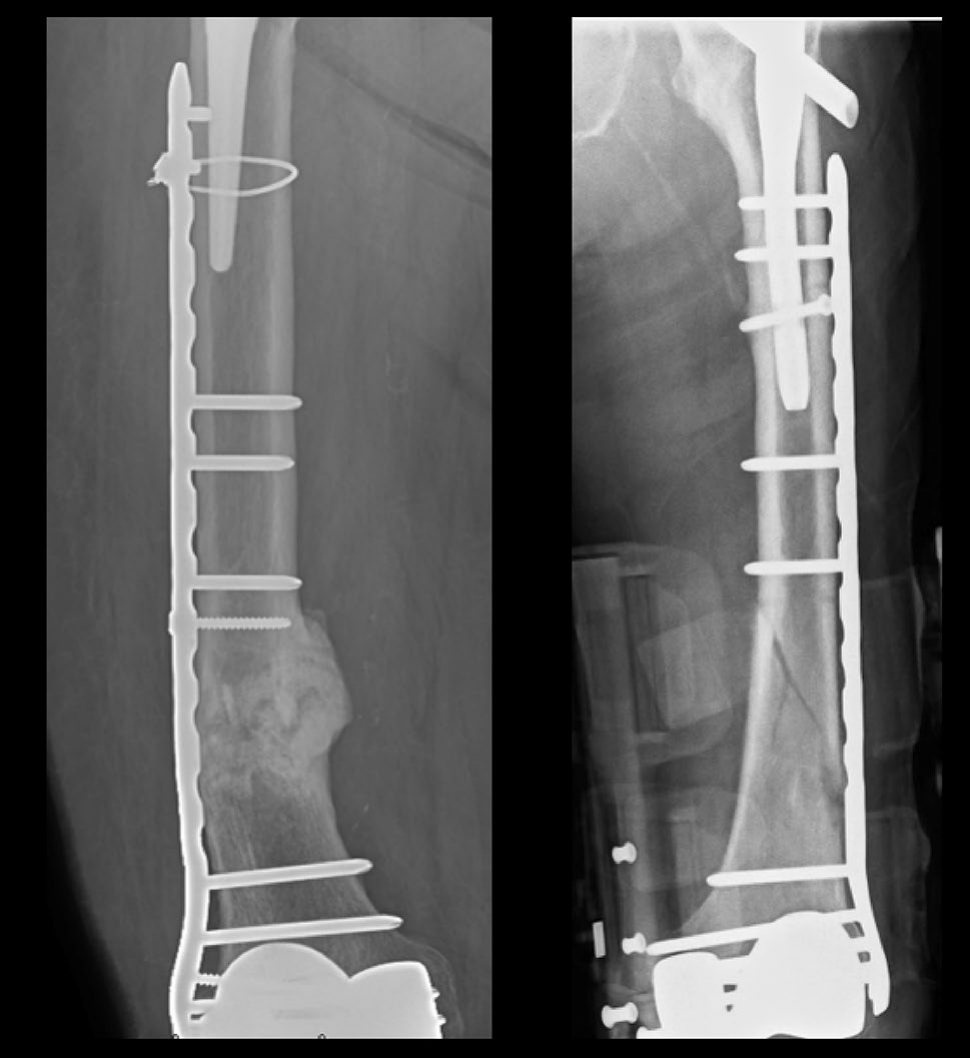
Post-operative radiographs of two patients who had peri-prosthetic fractures proximal to knee prosthesis, without the loosening of the prosthesis, managed by open reduction and internal fixation
